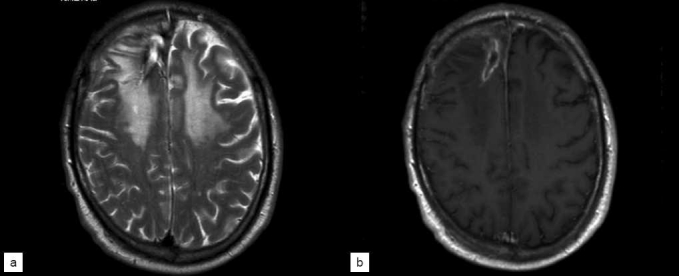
T2 (a) and T1 post-contrast (b) MRI obtained 6 months after craniotomy, demonstrating continued evolution of the right frontal necrotic lesion as well as bifrontal edema consistent with radiation effect
